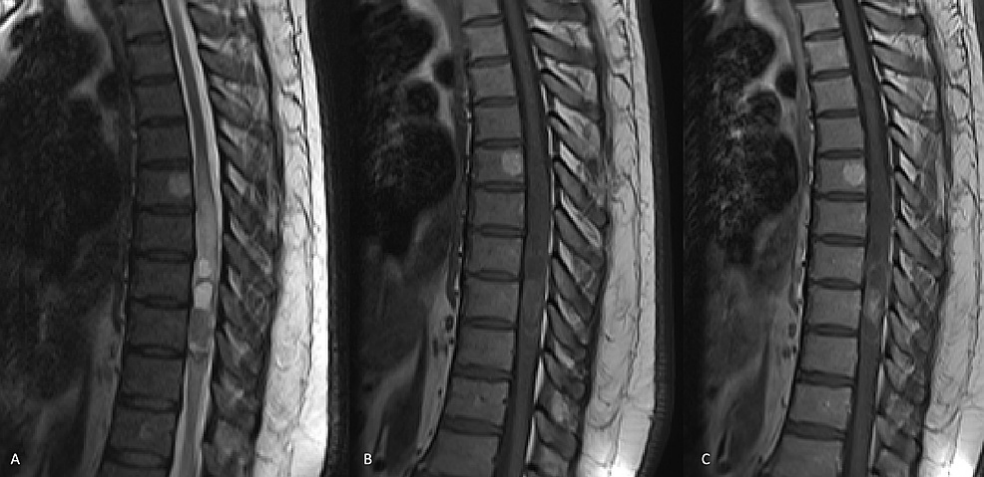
A thoracic spine MRI in a 48-year-old male with progressive myelopathy. Images in the sagittal plane in T2 (A), T1 without contrast (B), and T1 with contrast (C) demonstrating an enhancing intramedullary lesion in the T7-9 area with perilesional cord edema, a cyst, and a suggestion of a hemosiderin cap, the overall appearance of which was considered most likely to represent ependymoma. A hemangioma is incidentally noted in the T6 vertebral body. MRI: magnetic resonance imaging
